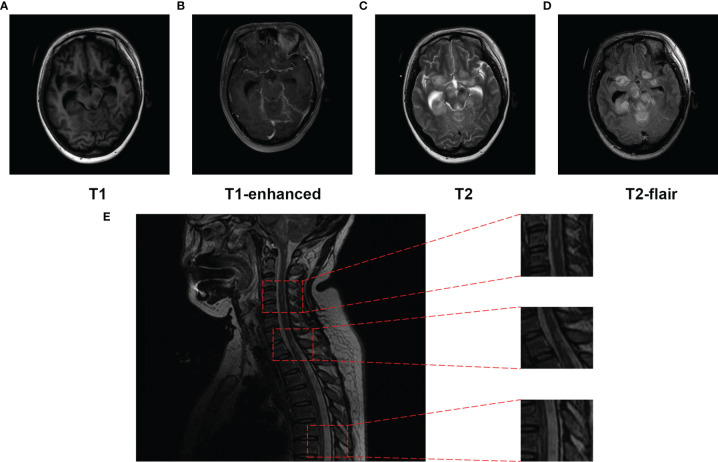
Magnetic resonance imaging (MRI) of the brain performed and spinal cord (T2) on day 15 post-hospitalization. (A–D) The frontal lobes, temporal lobes, ventriculus lateralis, brainstem, cisterna, and cerebellum were displayed as multiple patches, with high signal on T2WI, low signal on T1WI, apparently high signal on T2-Flair, and T1 enhanced. (E) Punctate and patchy lesions were presented as high T2 signals in the cervical and thoracic spinal cord.
